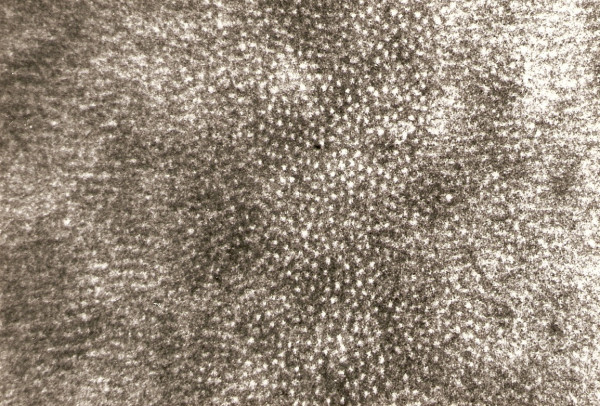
Figure-1 in higher magnification showing the advanced stage of tubular occlusion due to the superimposition of the x-ray image (mag. × 315).
